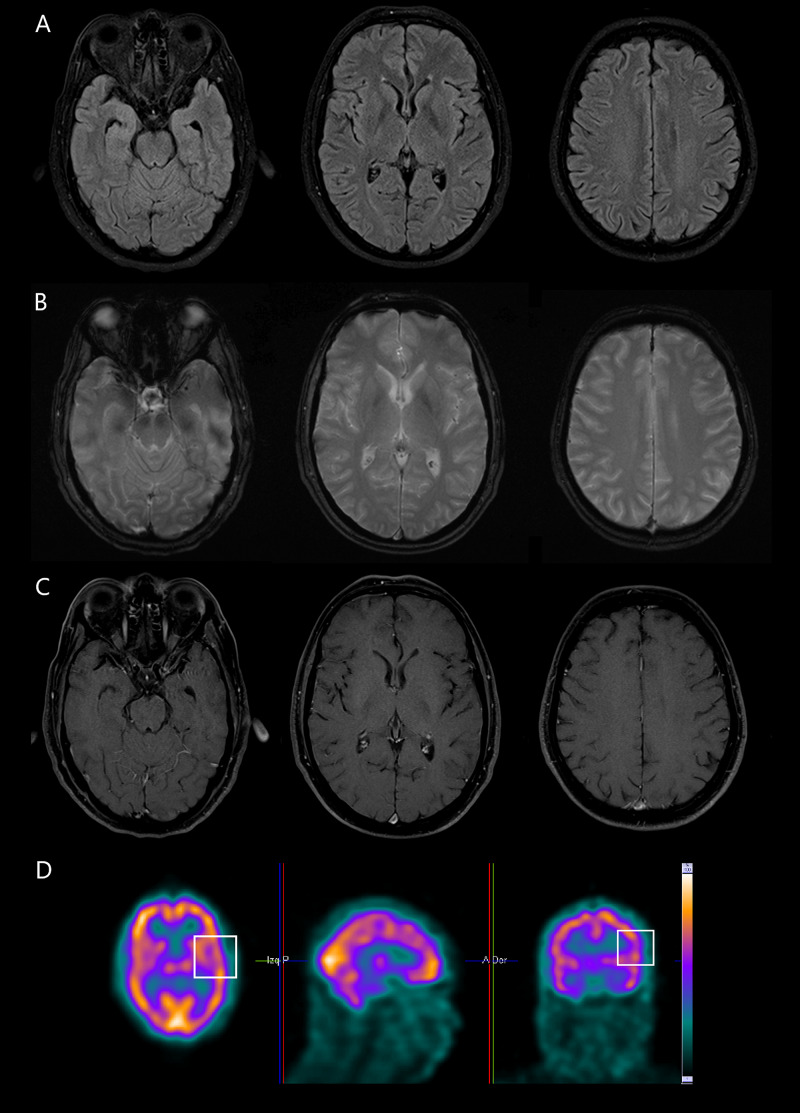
Brain MRI and SPECT. Brain MRI showing neither gray nor white matter lesions in T2-weighted-fluid-attenuated inversion recovery (panel A), lack of hemorrhages or microbleeds in susceptibility-weighted Imaging (panel B), and no cortical or meningeal enhancement in the contrast-enhanced t1-weighted image (panel C). Single-photon emission computed tomography shows a slight decrease in cerebral blood flow in the left frontotemporal cortex (white squares) (panel D). SPECT: single-photon emission computed tomography.
